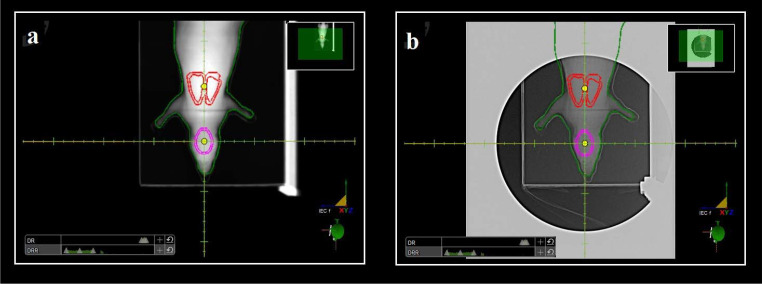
Screenshots of the anterior setup field with segmented external body (green), brain (purple), and lungs (red); (a) transferred from RayStation TPS to imaging software and (b) taken with on-board x-ray imaging before measurement for whole brain irradiation. Abbreviation: TPS, treatment planning system.
